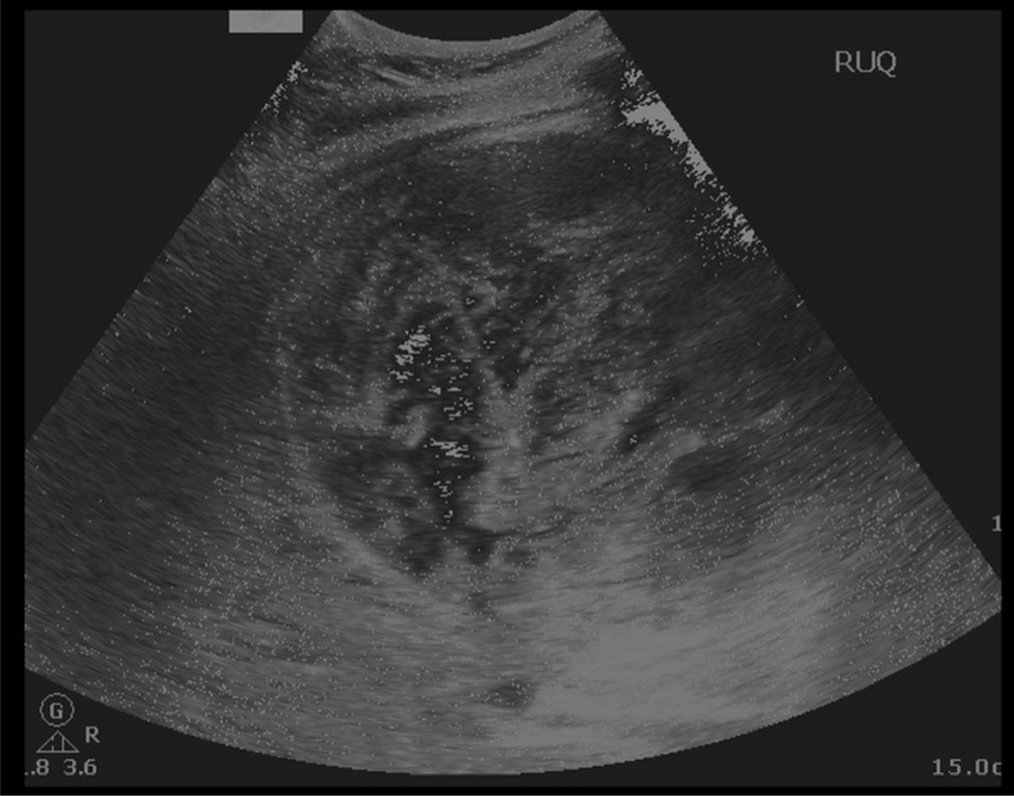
Ultrasound abdomen and pelvis
